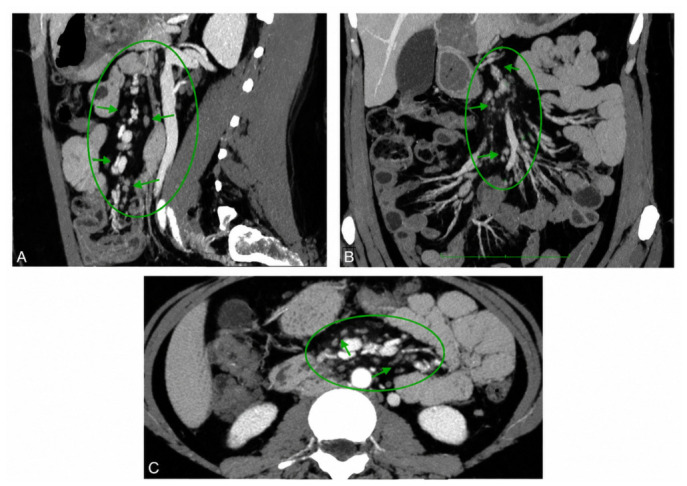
Contrast-enhanced computed tomography findings of mesenteric panniculitis. (A) Sagittal CT image demonstrating multiple sub-centimeter lymph nodes (green arrows) at the level of the mesenteric root. (B) Coronal CT image showing densification of the mesenteric fat with encasement of mesenteric vessels without direct invasion (green oval), and sub-centimeter lymphadenopathies (green arrows). (C) Axial CT image revealing diffuse hyperattenuating mesenteric fat infiltration (green oval) and associated lymph nodes (green arrows), suggestive of mesenteric panniculitis.
